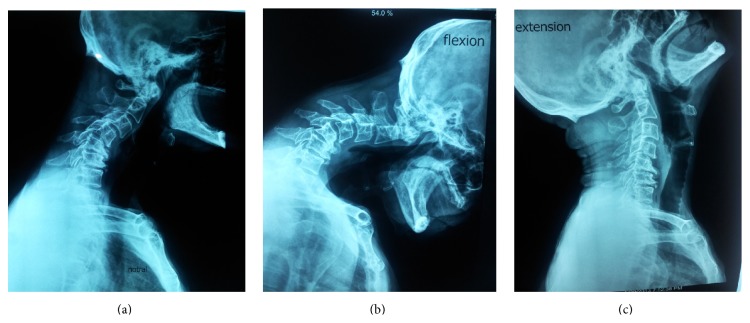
(a) Lateral cervical spine in neutral position in 2013, showing kyphotic spine with marked subluxation of C3 on C4 and C4 on C5 as well as degenerative changes at C5-C6 and C6-C7. (b) Lateral flexion radiograph. (c) Lateral cervical flexion and extension cervical radiographs indicating flexibility of the deformity.
